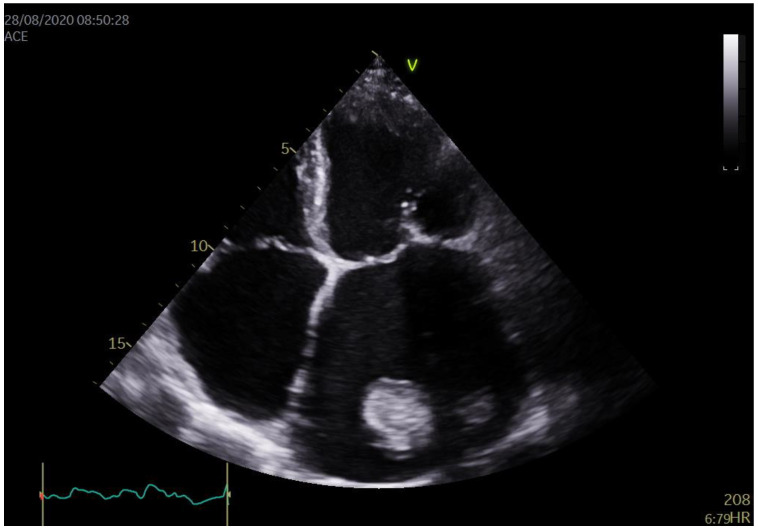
Echocardiography. Tumor formation in the enlarged left atrium (LA) (70 mm). One of the dimensions measured 53 × 40 mm, fixated by a wide base to the free wall of the LA.
